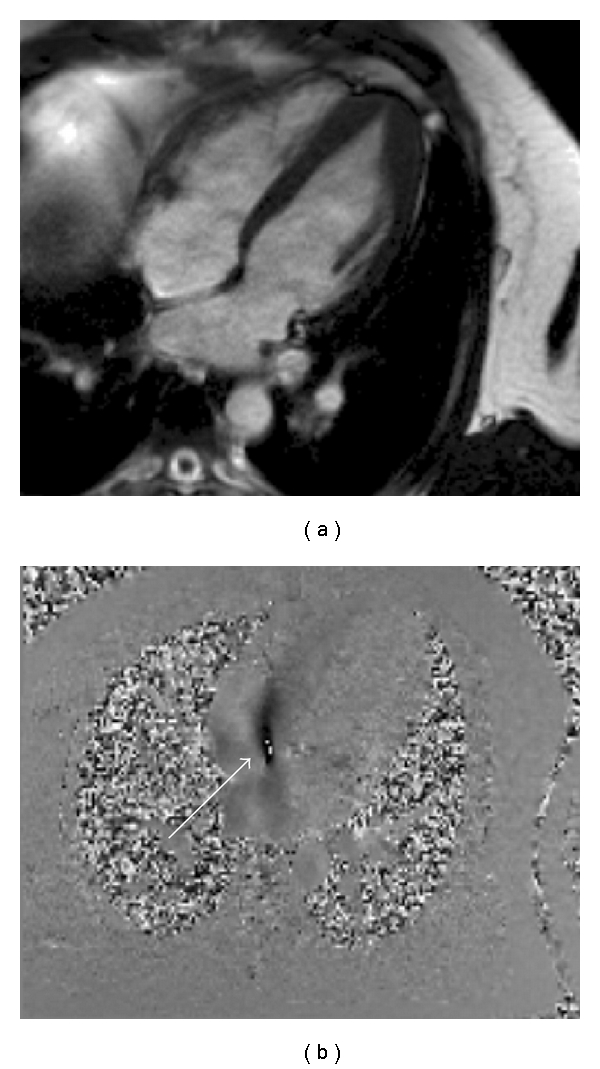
A patient with known apical HCM in whom transthoracic echocardiography had suggested a secundum ASD. (a) Cine 4 chamber image showing apical hypertrophy (b) flow sequence planned using the 4 chamber view shows flow across the intra atrial septum from left to right (arrowed), confirming the diagnosis. In this case, although the right heart does not appear dilated, the Qp : Qs was calculated as 2 showing the presence of a significant shunt.
